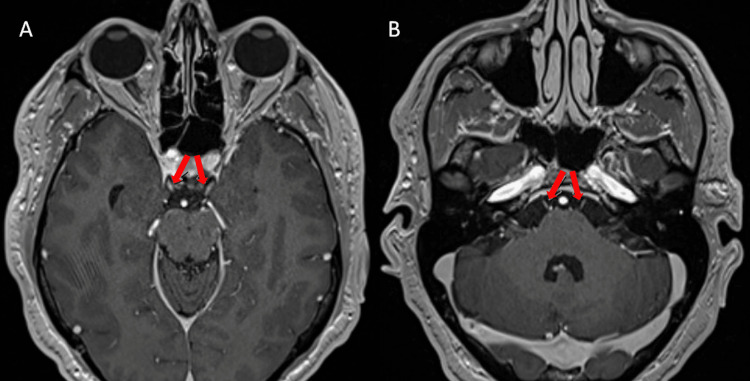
MRI head with contrast images MRI head with contrast shows enhancement of cisternal segments of bilateral cranial nerves III (A) and VI (B)
